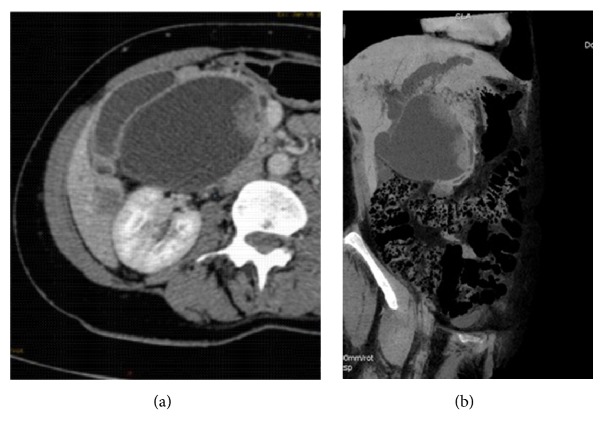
CT sections after injection of iodized product at portal venous phase. (a) Axial section: cystic mass with secondary lesion of the VI segment. (b) Oblique reconstruction showing mass relationship with the bile ducts.
